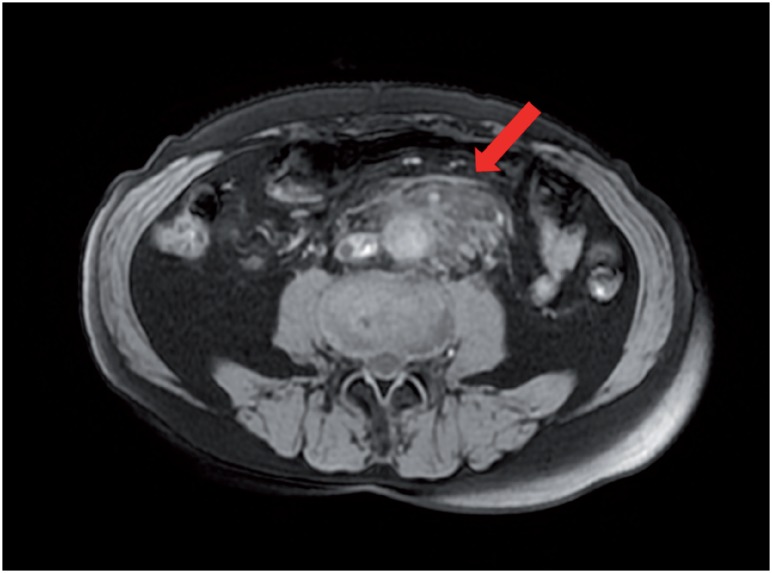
C) Magnetic resonance imaging demonstrated rpf masses as low and high intensity (according to inflammation) on the T2-weighted images.
